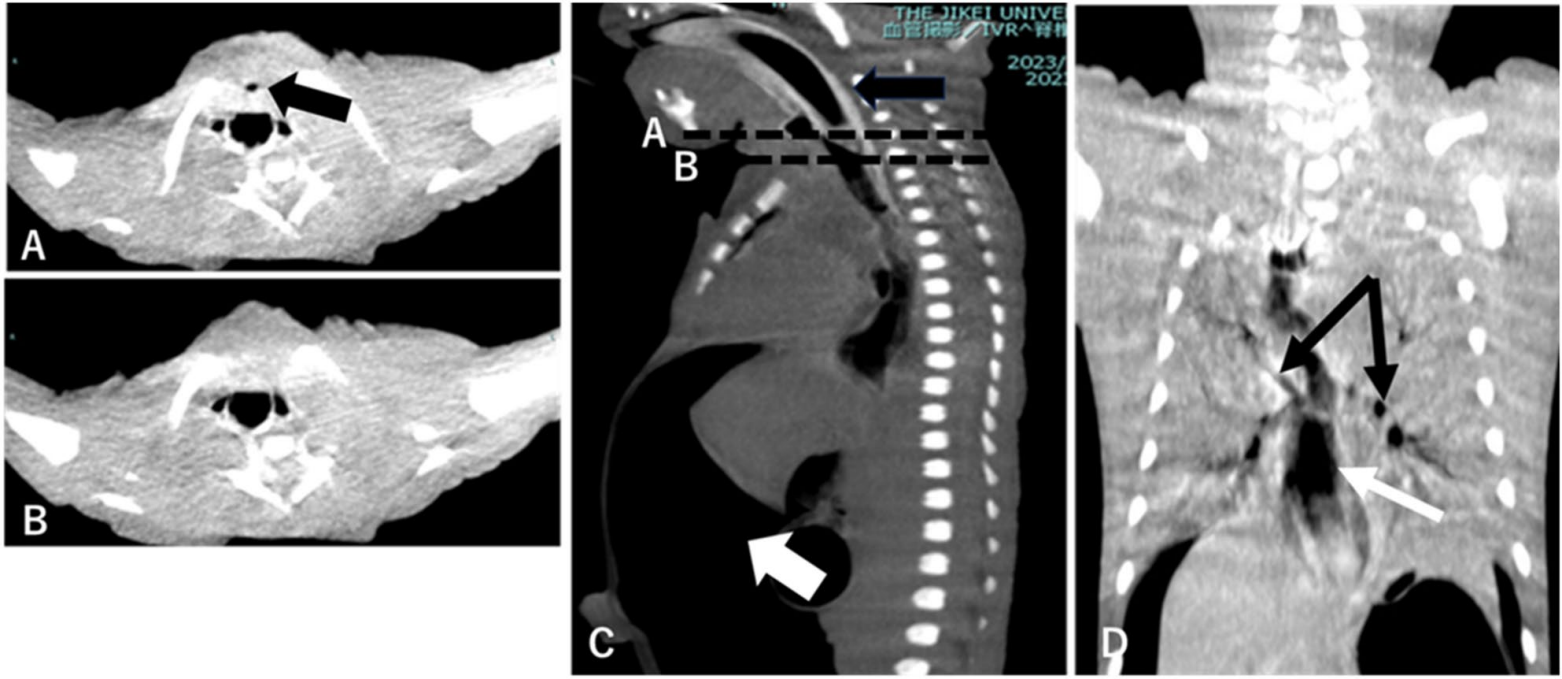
༎ Neck and chest computed tomography. A Axial neck computed tomography at the level indicated by line A on the sagittal view C. The black arrow indicates the blind-ending upper airway subglottic pouch. B Axial neck computed tomography at the level indicated by line B on the sagittal view (C), demonstrating absence of a patent tracheal lumen. C Sagittal cervicothoracic computed tomography. The black arrow shows the supraglottic airway in situ; the white arrow indicates a large volume of free intraperitoneal air. D Coronal cervicothoracic computed tomography demonstrating bilateral main bronchi (black arrows) and markedly dilated esophagus (white arrow), consistent with gas insufflation
